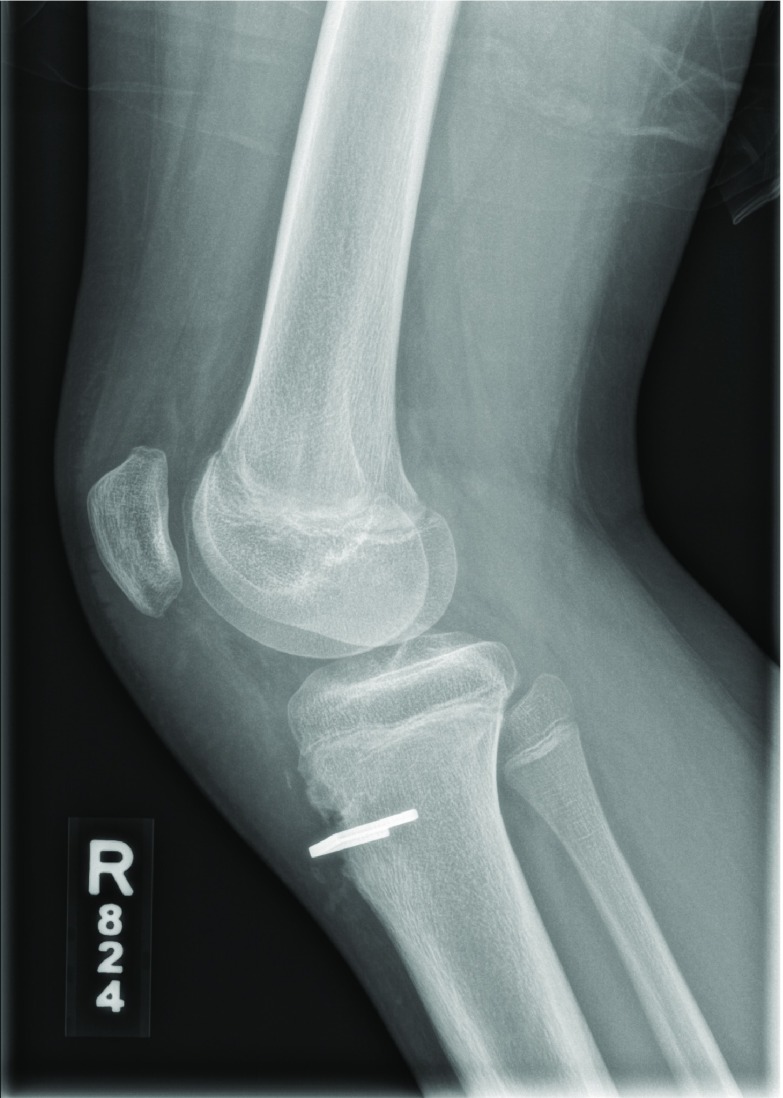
Lateral postoperative radiographs of the patient described in Case 1.
